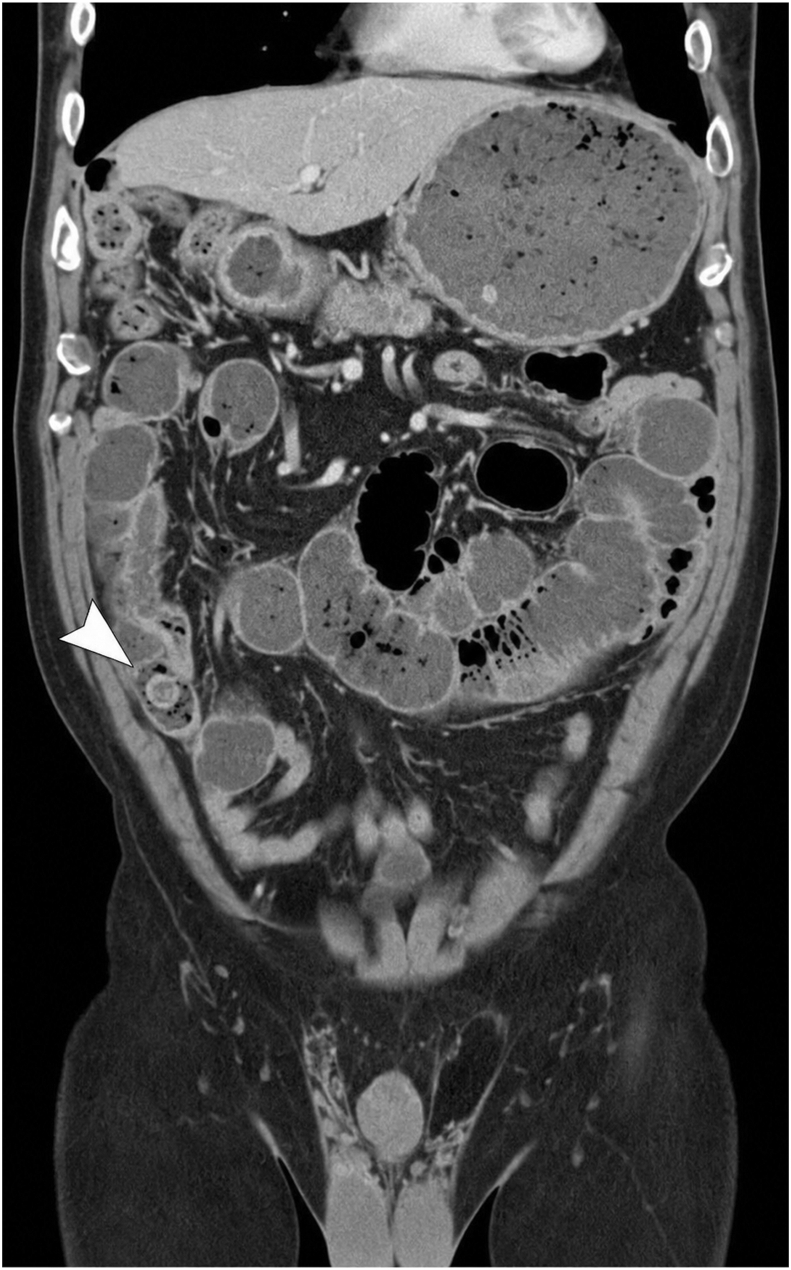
Abdominal computed tomography coronal view showing dilated proximal small bowel loops and a transition point in the right lower abdomen caused by a rim-calcified intraluminal lesion (arrowhead).
